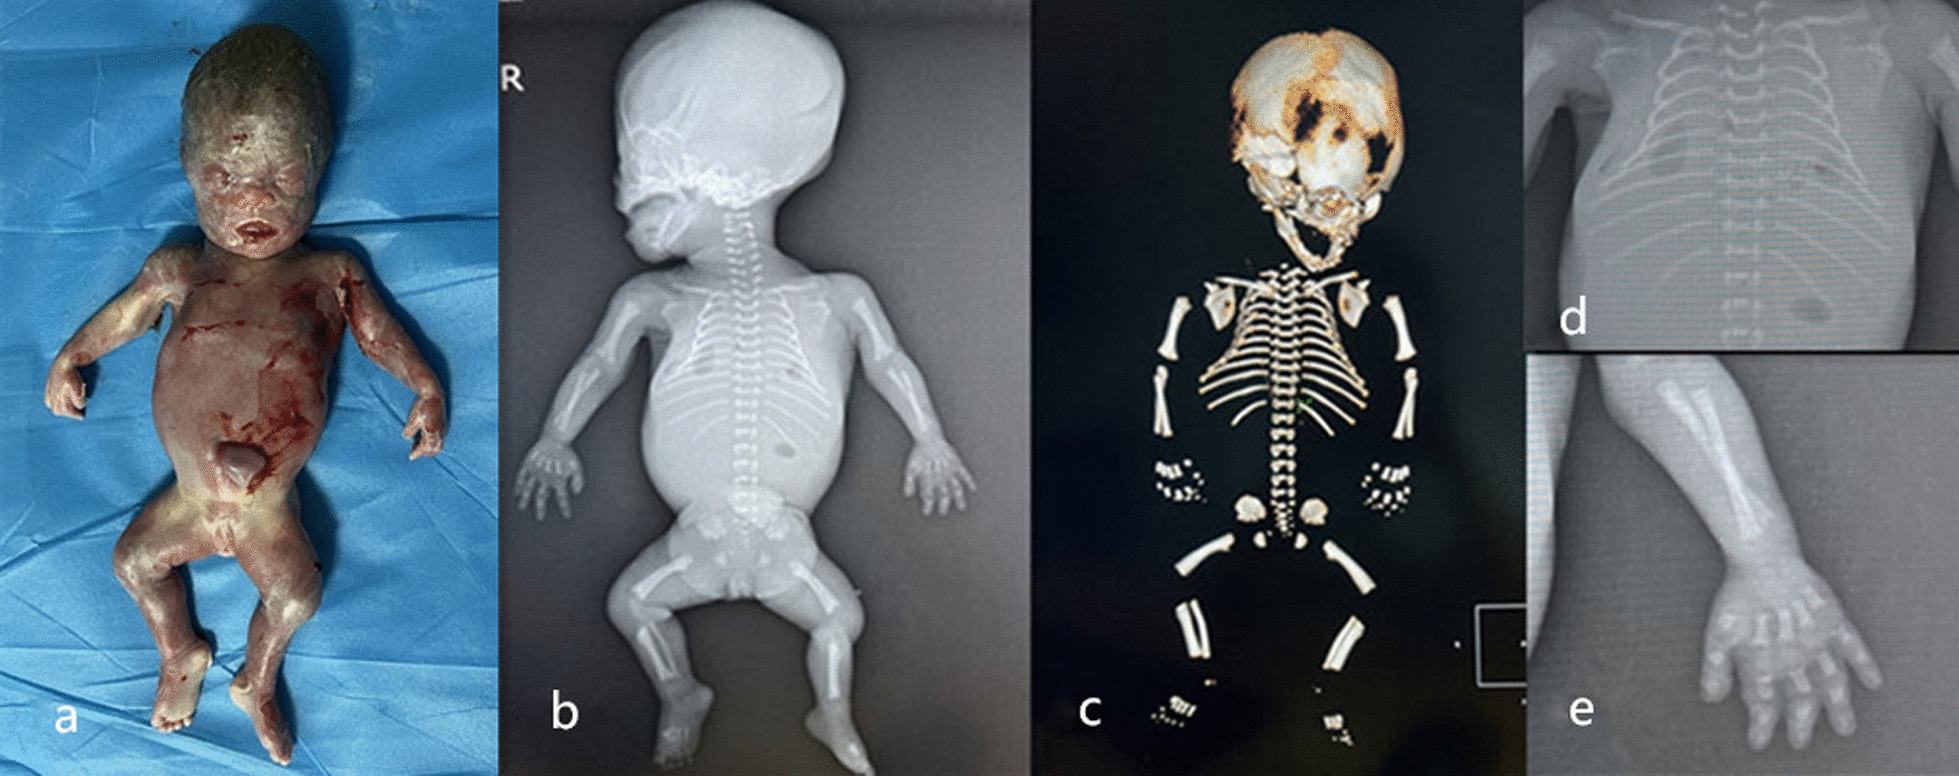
Physiological characteristics and radiographic evidence of the corpse. a Physiological characteristics of the corpse: disproportionate shortening of long bones, frontal bossing, midface hypoplasia, and protuberant abdomen, talipes equinovarus in the right side. b X-ray image showing disproportionate shortening of long bones, large skull roof and small skull base. c Computerized tomography 3D bone reconstruction showing narrow thoracic shape as a bell, flat midface and spine. d X-ray image showing narrow chest and flat vertebrae. e the trident hand
